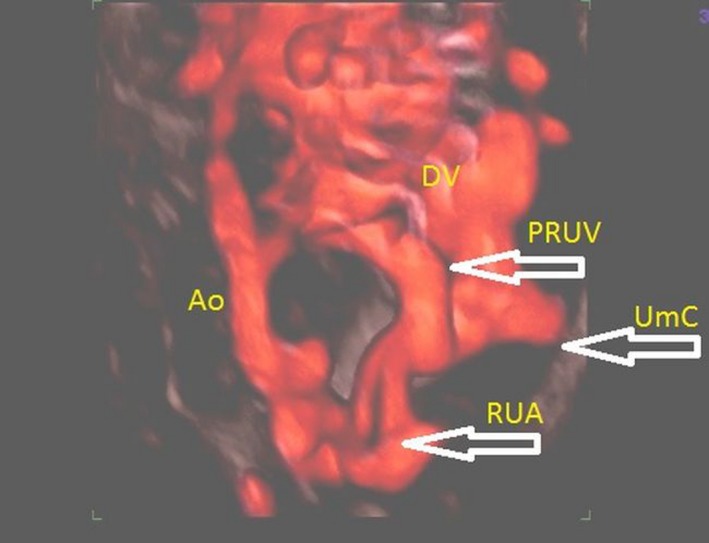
Persistent right umbilical vein (PRUV) in 3D power Doppler imaging of a fetal venous system. DV, ductus venosus; PRUV, persistent right umbilical vein; RUA, right umbilical artery; UmC, umbilical cord; Ao, aorta.
